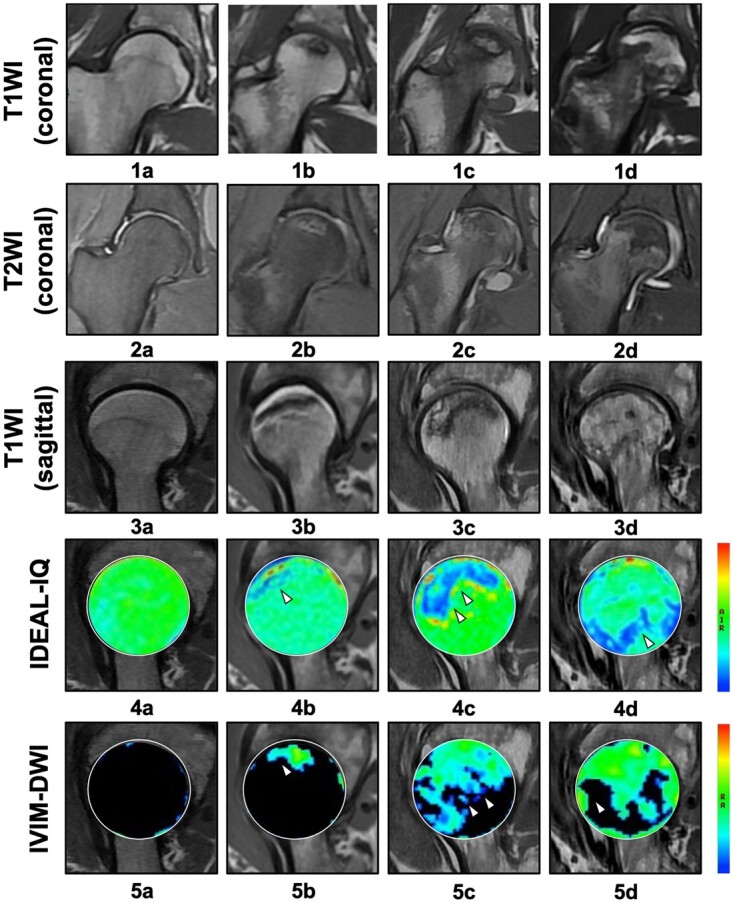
The progression of ARCO stage and signal changes in sagittal/coronal T1WI, sagittal T2WI/*f*s, sagittal IDEAL-IQ and sagittal IVIM-DWI sequences. (a1–a5) A 52-year-old female participant without SIONFH exhibited an even color gradation of the femoral head and clear boundaries at imaging. (b1–b5) A 53-year-old male ARCO Stage I patient showed a small blue band and a green color spot in the IDEAL-IQ and IVIM-DWI images, respectively. (c1–c5) A 61-year-old female ARCO Stage II patient exhibited mixed-color bands in both techniques. (d1–d5) A 41-year-old male ARCO Stage III patient showed a blue band indicating the penumbra area in IDEAL-IQ and diffuse and mixed colors (green–yellow–red) in IVIM-DWI.
